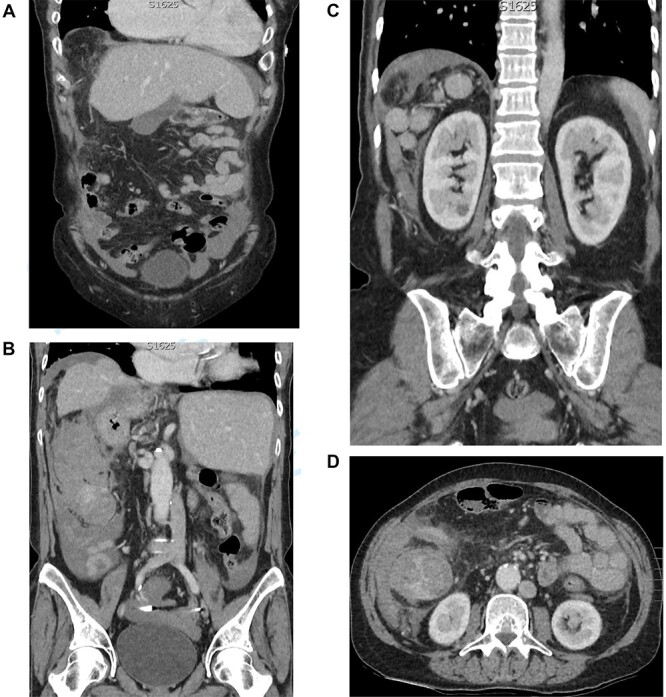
(A) CT coronal cross section demonstrating a left-sided liver and gallbladder. (B) CT coronal cross section demonstrating a right-sided stomach and abdominal aorta, left-sided inferior vena cava and the heterogenous lobulated mass with free fluid. (C) CT coronal cross section demonstrating the five splenunculi. (D) CT axial cross section demonstrating the 130 mm heterogenous lobulated mass in the right abdomen
